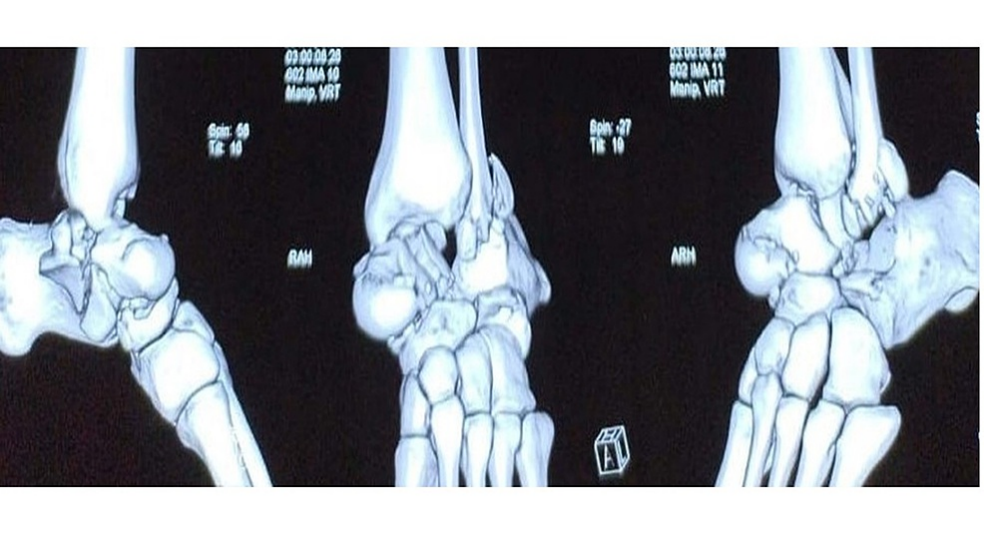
Preoperative CT scan showing Pan-talar dislocation
